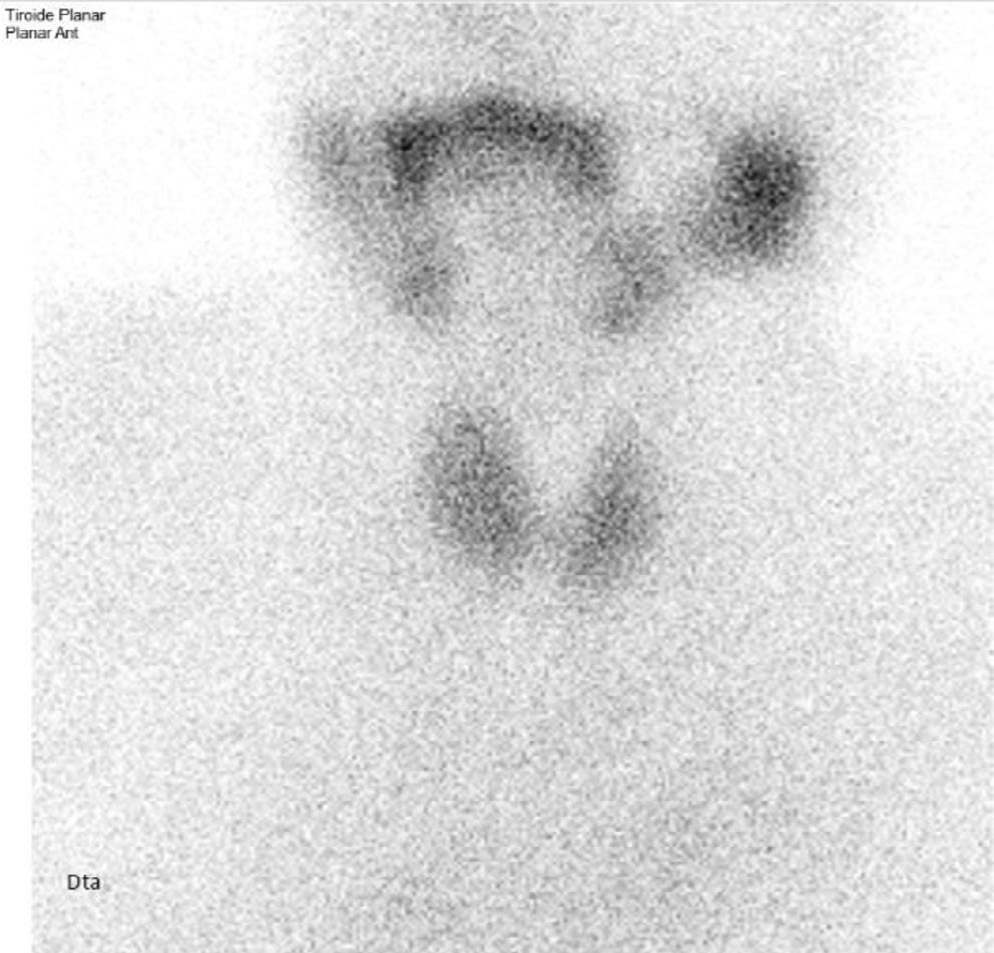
^99m^Tc‐pertechnetate thyroid scintigraphy (coronal view); globally preserved uptake with no hyperactivity areas suggestive of focal hyperfunction (report highlights possible interaction of amiodarone in uptake pattern up to 2 years after withdrawal).
